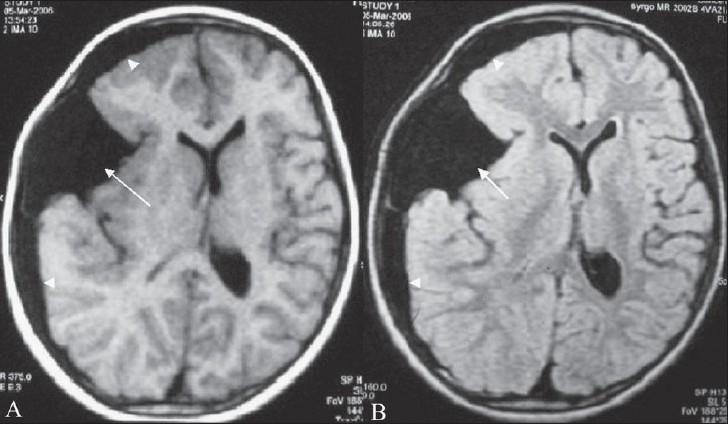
Axial T1W (A) and axial FLAIR (B) images show a hypointense arachnoid cyst (arrow) and an associated subdural hygroma (arrowhead)
